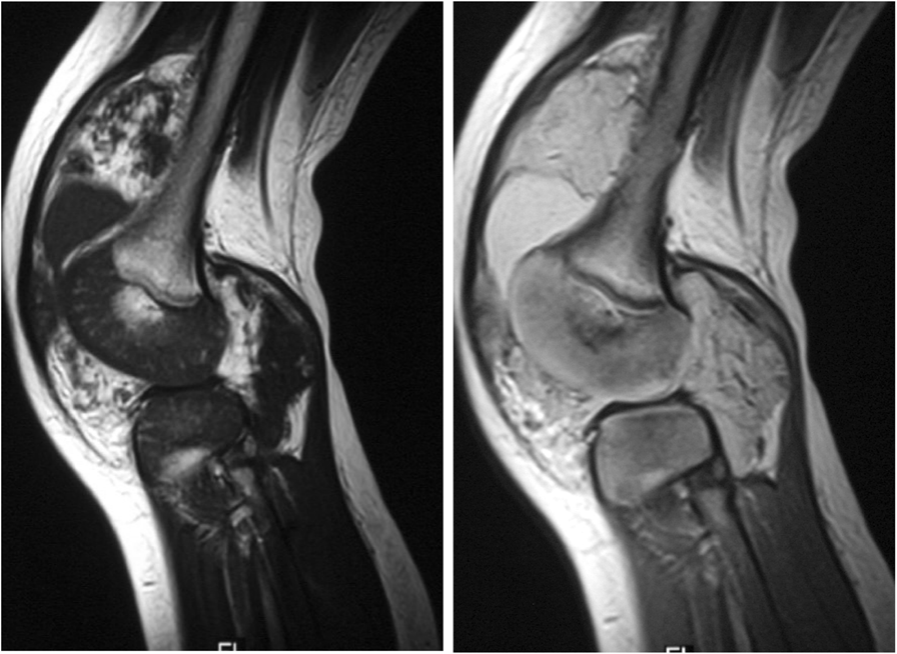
Knee MRI of patient 1. Sagittal T1 and T2 -weighted images show the extension of the IAVM in the synovial space
